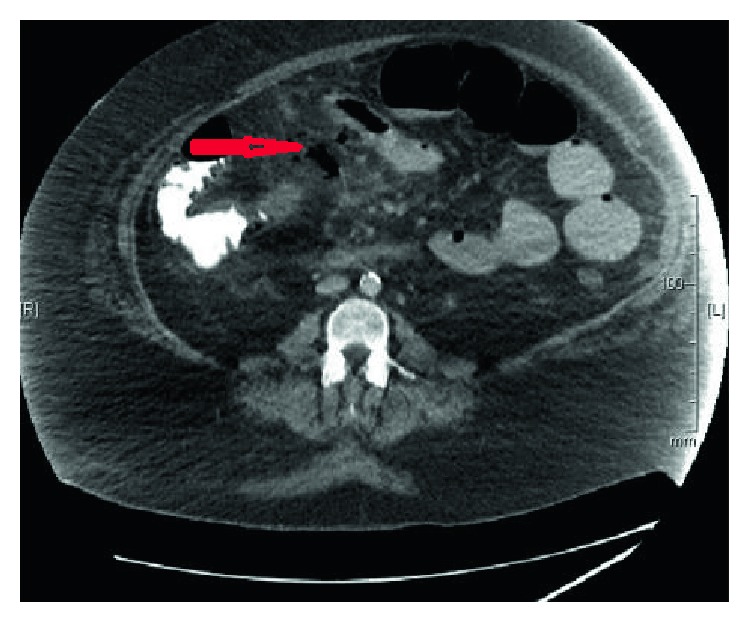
CT contrast scan depicting free air in pocket of abscess where bowel was perforated (short red arrow).
